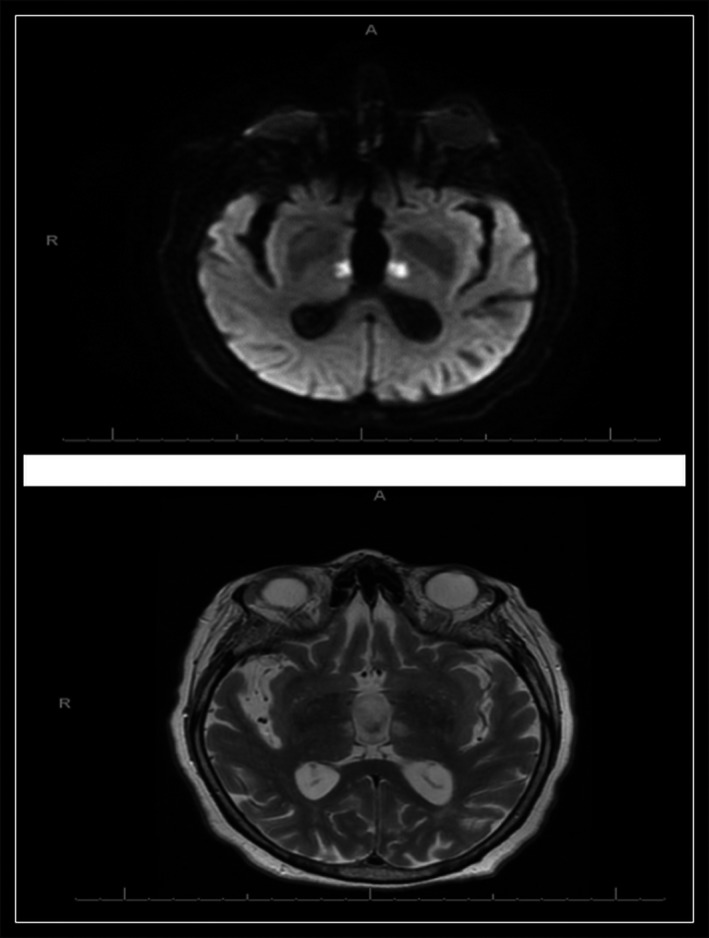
Diffusion‐weighted magnetic resonance imaging showing acute bilateral thalamic stroke.
